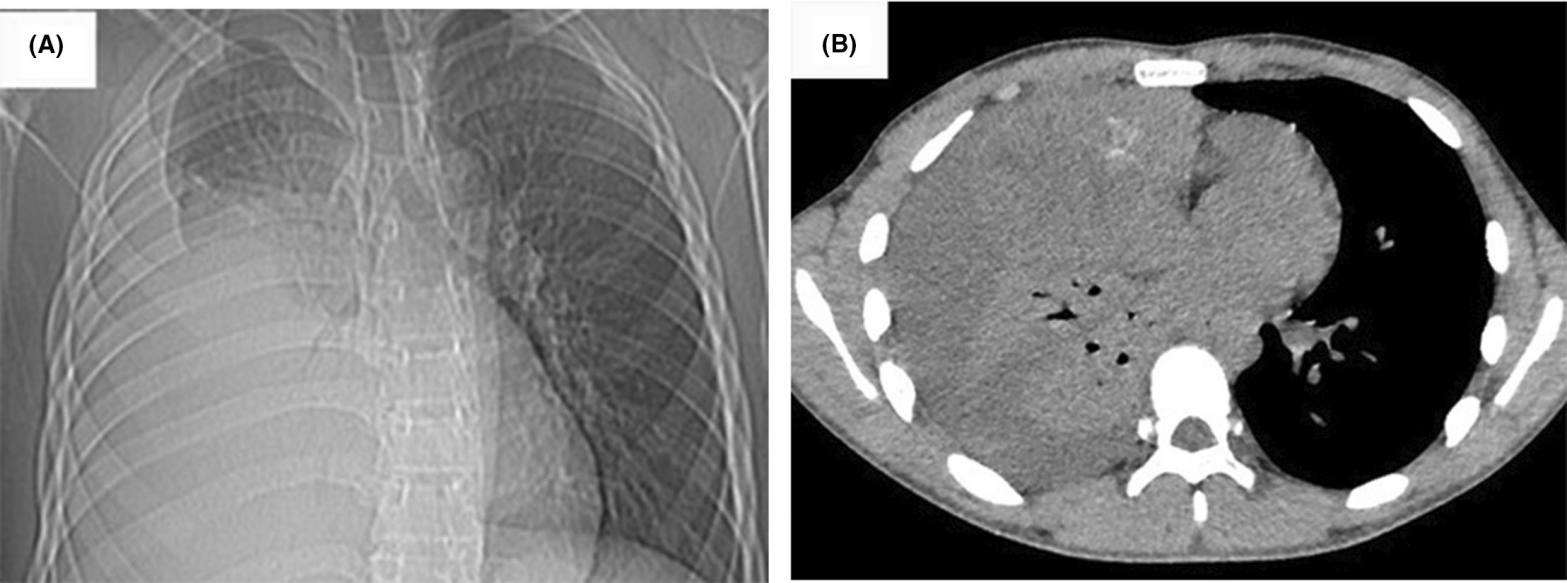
(A) Anterior–posterior chest x‐ray and (B) computed tomography scan of the chest: a huge tumor of mediastinum and severe right pleural effusion were first discovered.
